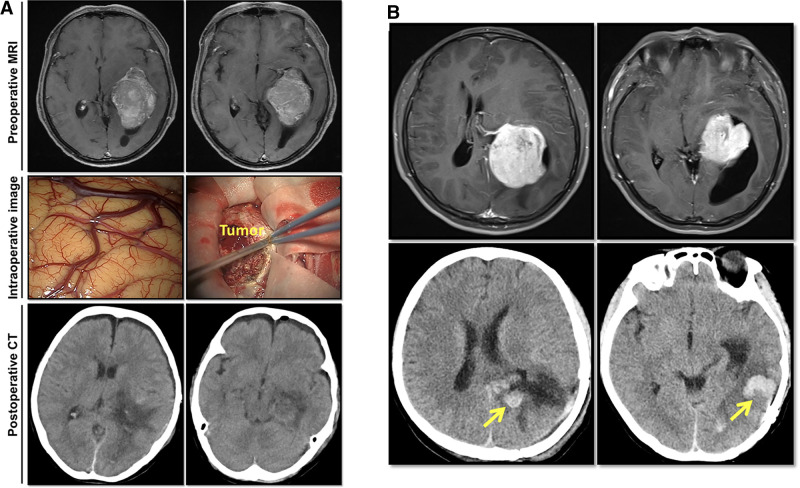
Imaging studies of two representative lateral ventricular trigone meningioma (LVTM) patients. (A) A 63-year-old female patient presented with dizziness and headache. Preoperative axial enhanced T1-weighted magnetic resonance imaging (MRI) showed a huge mass in the left trigone area. Intraoperative observation and postoperative computed tomography (CT) imaging showed a total tumor resection. On a 6th postoperative day, she had a fever of 38.5°C with a headache and meningeal signs. The CSF test showed elevated white cell count (394 10^6^/L), protein level (>3,000 mg/L), and decreased glucose level (<1.1 mmol/L). Lumbar drainage was performed, and meropenem was used according to empirical therapy. Due to the negative CSF smear and culture results, meropenem was used continuously. On the 20th postoperative day, the patient had maintained a normal temperature for a week. CSF tests were repeated three times with normal results. The drainage was removed, and the meropenem was stopped. The patient was discharged from the hospital on the following day. (B) A 35-year-old male patient presented with a headache. Preoperative axial enhanced T1-weighted MRI revealed a large mass in the left trigone area (upper image). Postoperative 24-h CT showed total tumor resection with the tumor cavity hemorrhage (lower image). The patient showed mild headache without fever disturbance of consciousness. On a postoperative day 7, he had a continuous fever of 39°C. The CSF test showed elevated white cell count (754 10^6^/L), protein level (>3,000 mg/L), and decreased glucose level (<1.1 mmol/L). Lumbar drainage was placed, and meropenem was used according to empirical therapy. As the CSF culture showed methicillin-resistant *Staphylococcus aureus*, meropenem was replaced by vancomycin. On the 33rd postoperative day, the patient had maintained normal temperature for a week with 3 times of normal CSF tests. The drainage was removed, and vancomycin was stopped. The patient was discharged from the hospital on the following day.
